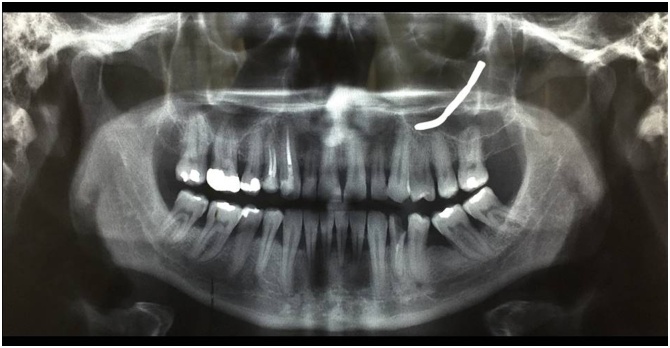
The dental X-ray evidenced the “presence of a metal prosthesis located in the left side of the facial skeleton and the absence of areas of apical/radicular osteolysis affecting the teeth”.
